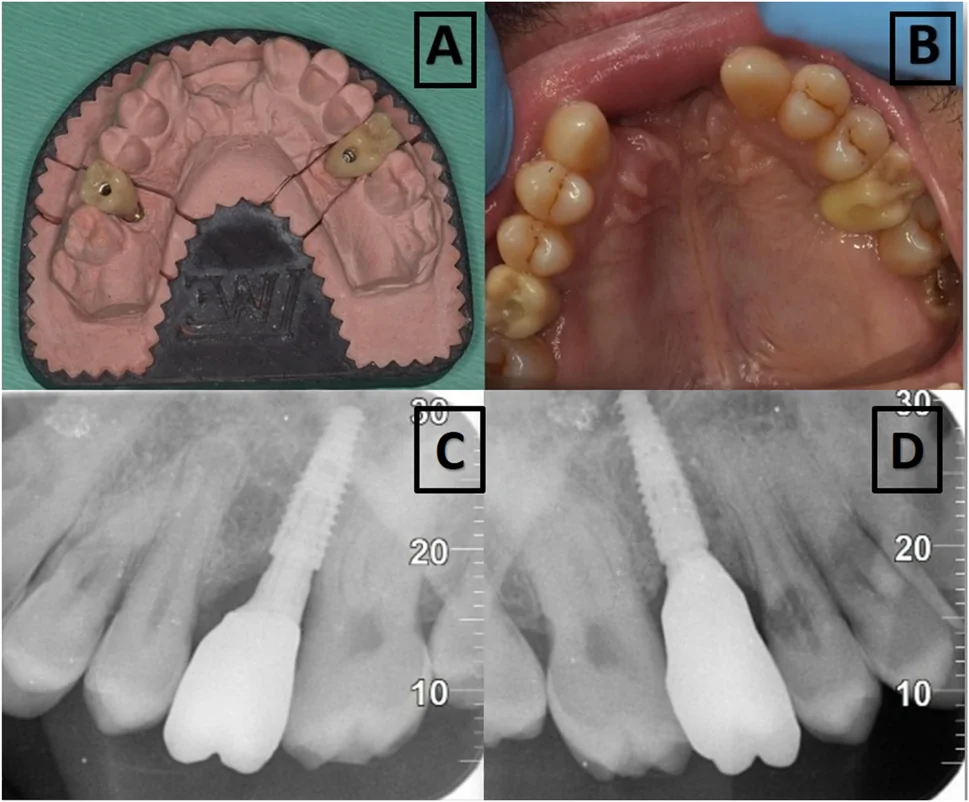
A Final crowns on laboratory model. B Clinical view after screw-retained crown placement. C, D Periapical radiographs showing precise fit of the final crowns with no detectable peri-implant bone loss
